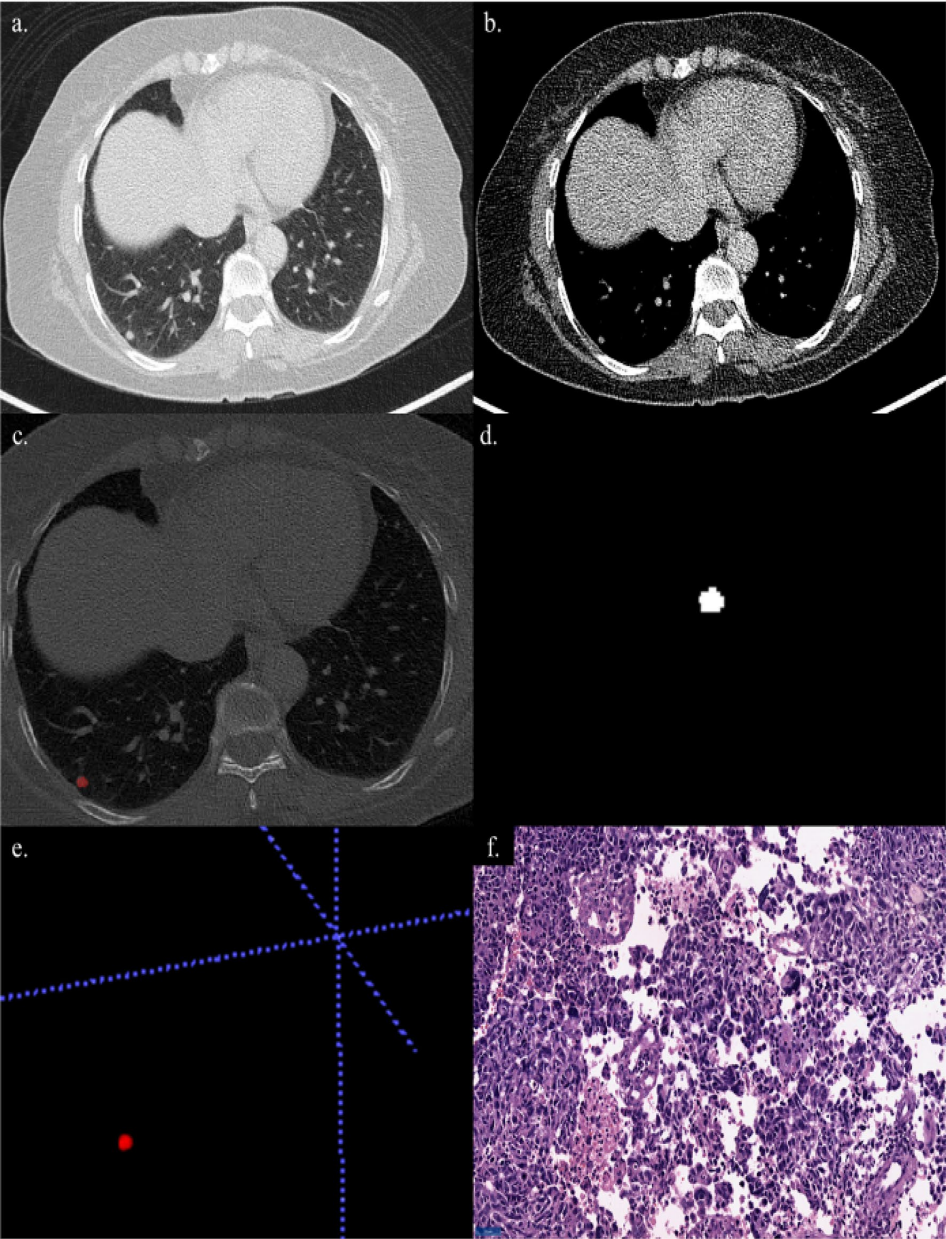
A 66-year-old female with a lump in the right breast. (a, b) Axial sections of CT thorax showing a nodule in the posterio-basal segment of the right lower lobe. (c) Creation of ROI for segmentation. (d) 2D-segmented nodule. (e) 3D-volumetric rendering of the nodule. (f) A final diagnosis—metastasis HPE: Primary malignancy—carcinoma breast.
